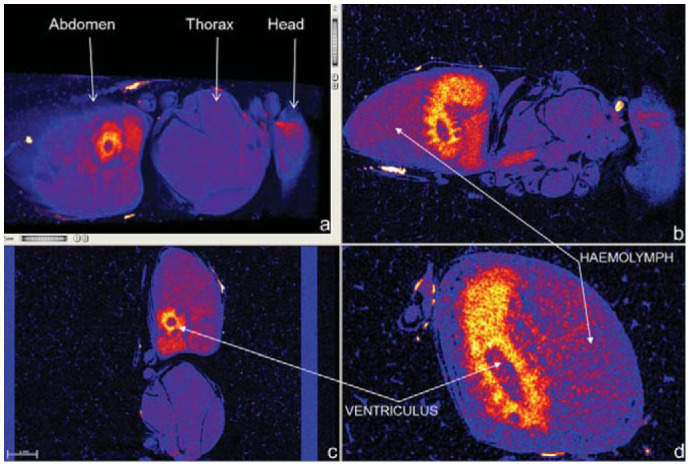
A 3D volume rendered image with BeeView software of a live honey bee showing the three body segments (a) and orthogonal, 2D images (b, c, and d) showing the passage of radiographic contrast from the ventriculus (true stomach) to the haemolymph in the coelum. Images were rendered 1.5 hours after ingestion of contrast. High quality figures are available online.
